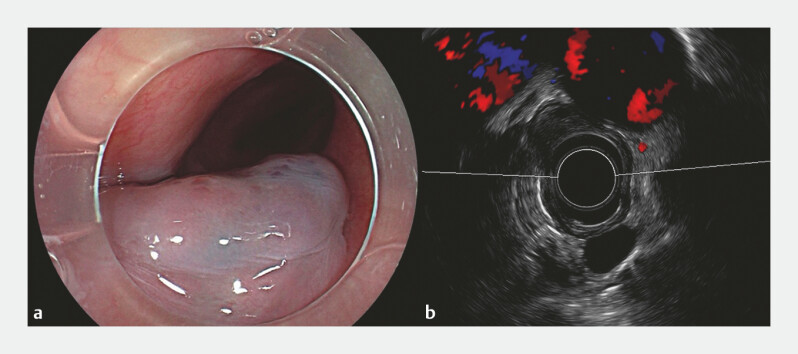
Colonoscopy and endoscopic ultrasound.
